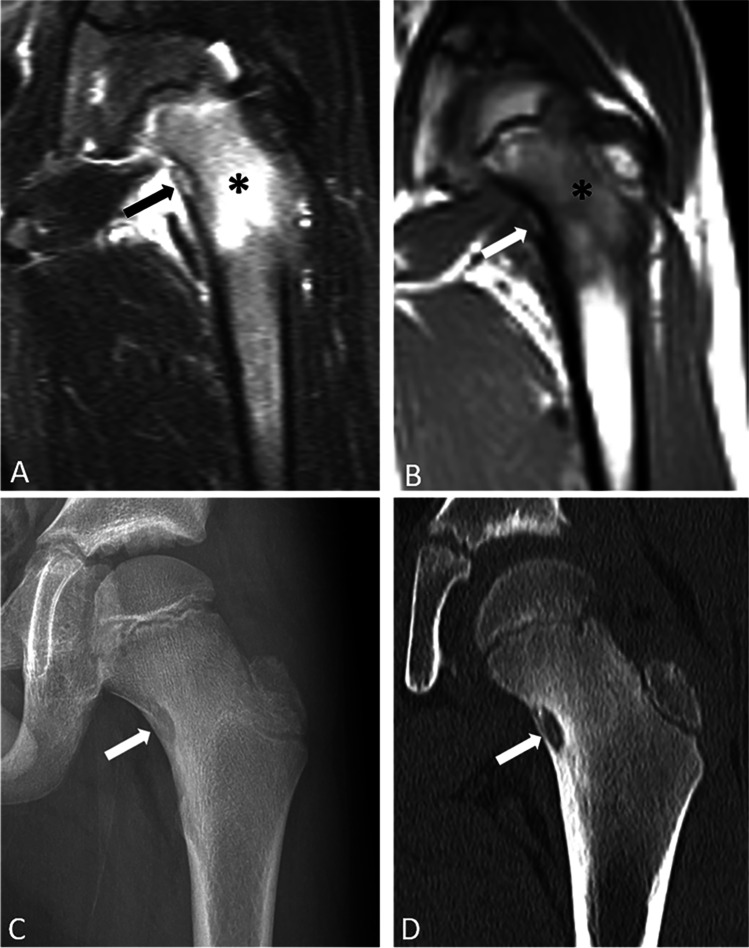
A 10-year-old male was referred with a working diagnosis of osteosarcoma. Coronal T1 (A) and STIR (B) MR images show a large joint effusion and increased T2 signal in the neck and intertrochanteric region of the proximal femur that is out of proportion to the findings on T1 where there is relative preservation of marrow fat signal (asterisk). Recognizing a bone marrow edema pattern, closer inspection of the MRI images reveals an obvious round to ovoid lesion in the femoral neck medially (arrow), that was missed given the lack of consideration of the diagnosis on the differential. Subsequent AP radiograph (C) and coronal CT (D) confirm the diagnosis of an intracortical OO (arrow)
